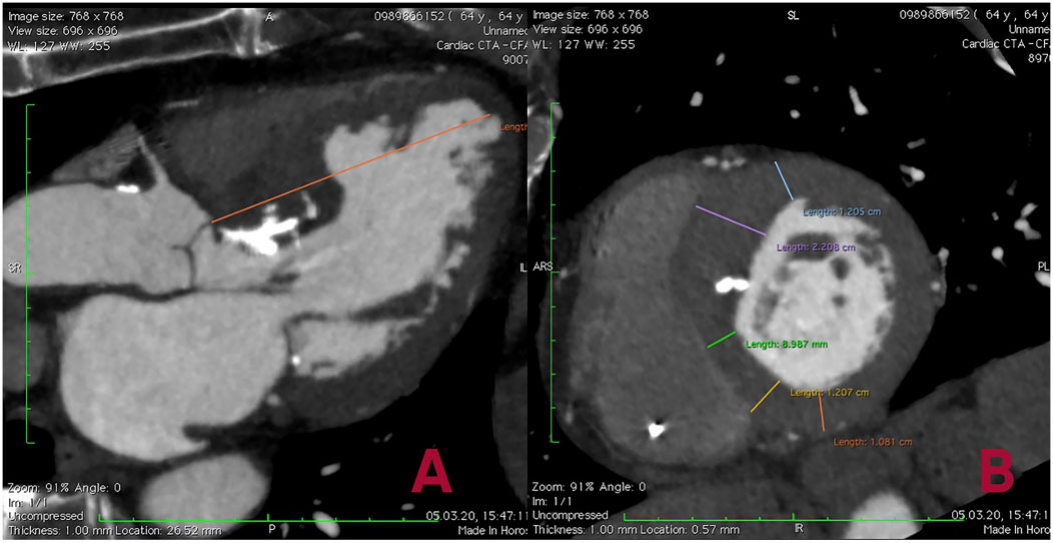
Preoperative computed tomography planning using long- (A) and short-axis (B) views, where the distance from the aortic valve ring to the left ventricular apex in the 3-chamber view and the thickness of the left ventricle and interventricular septum are measured at a distance of 8 mm from the aortic valve ring at the end of diastole.
